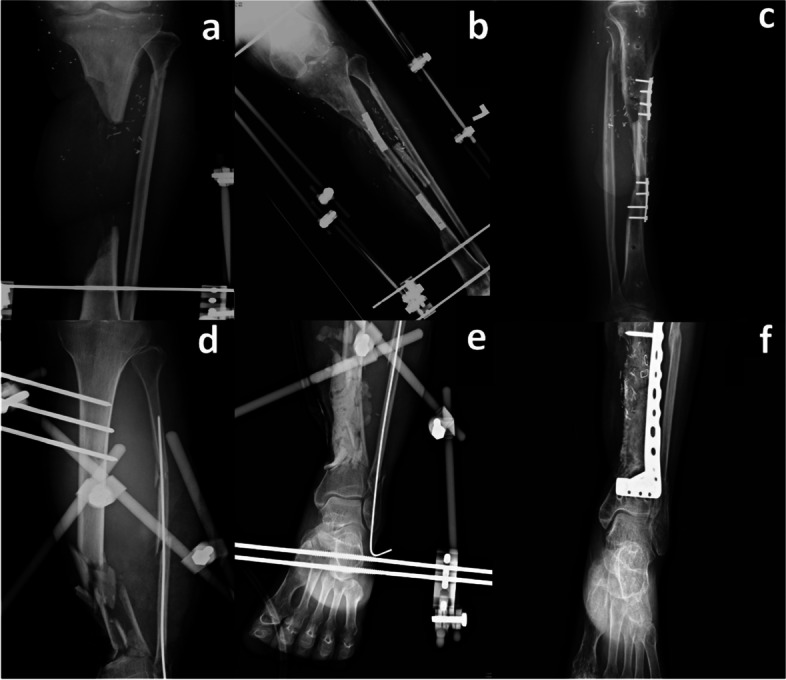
Radiographic images of pre-, post-operative, and united IIIC fractures. a d Preoperative tibial fractures with temporary external fixators; b e Postoperative images: b vascularized bone graft fixed with plate, e Masquelet stage I with cement spacer; c f United tibial fractures
